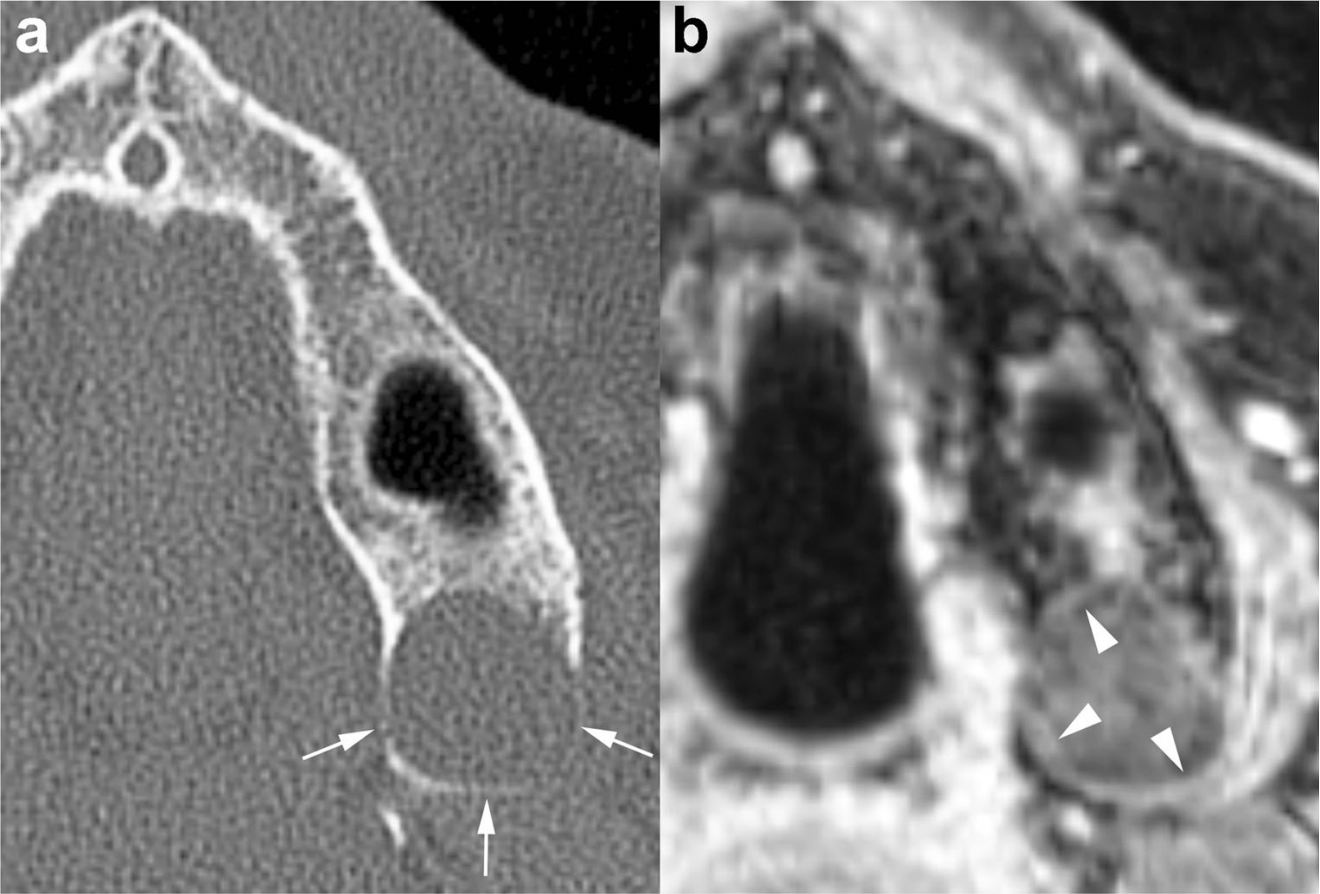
Unilocular OKC in the left maxillary tuberosity. a Axial MDCT image with bone window demonstrates remodelling and thinning of the adjacent cortices (*arrows*). b Note thin rim enhancement within the lesion on enhanced T1-weighted fat-saturated sequence (*arrowheads*)
